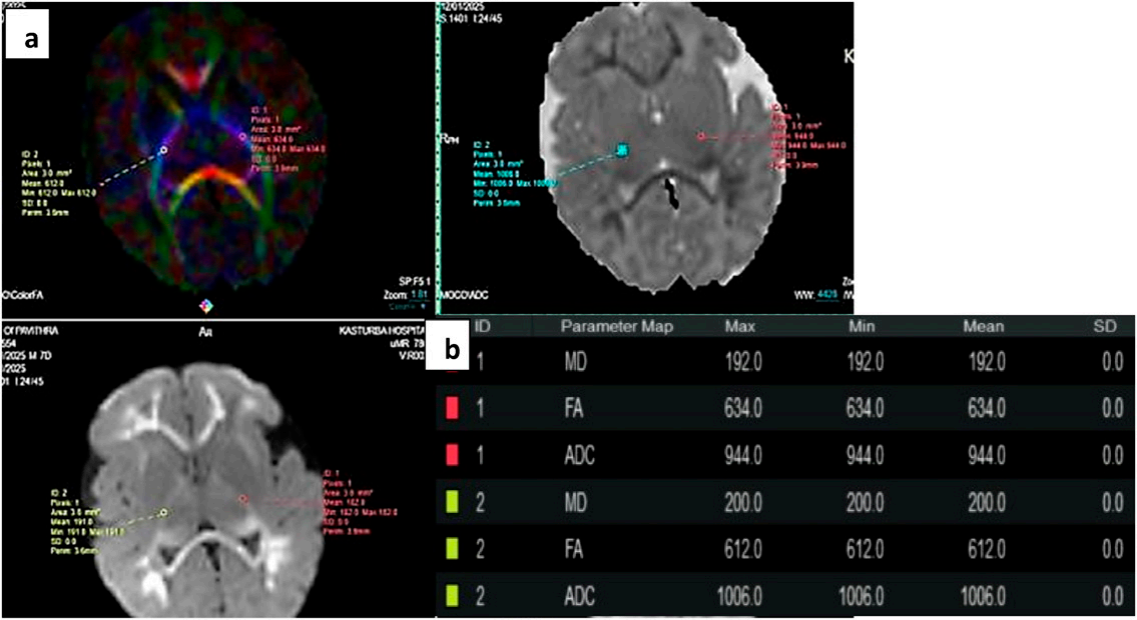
(a) shows ROI placement in the posterior limbs of internal capsules in Color FA maps bilaterally with corresponding DWI and ADC maps. (b) shows the FA, ADC and MD values in the ROIs.
